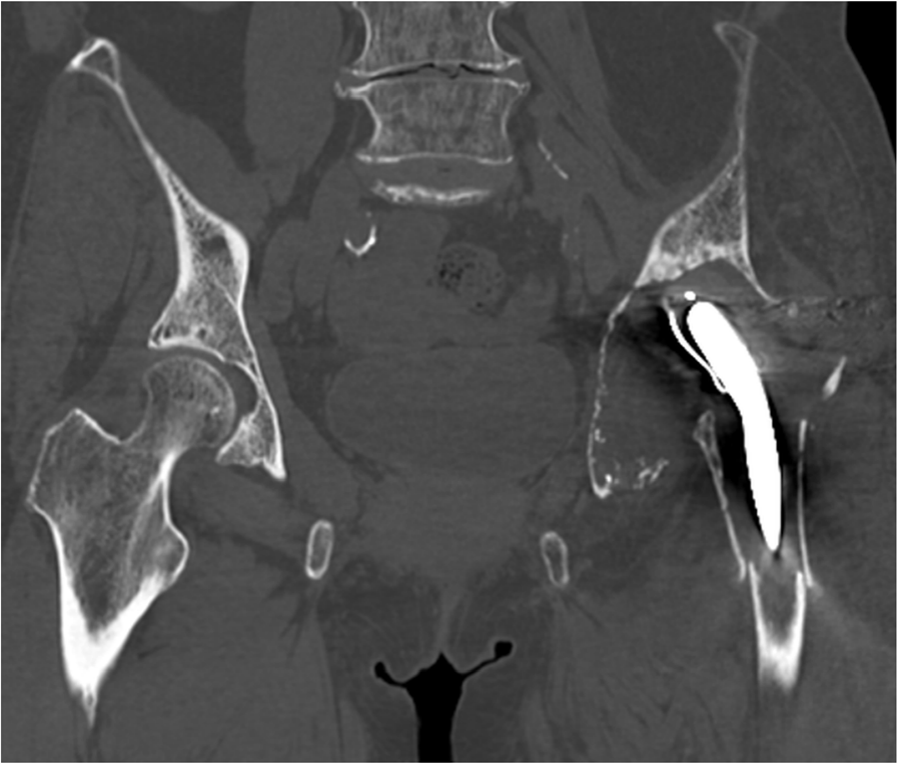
A plain computed tomography image of the bilateral hip in the coronal section showing a notably flimsy inner table of the left acetabulum. The periprosthetic fracture of the left femur can also be seen in this view
